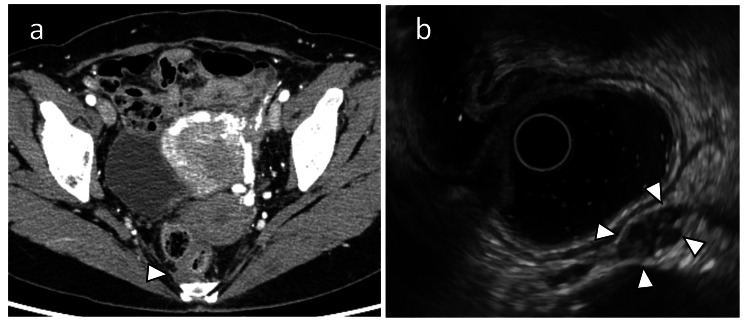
Preoperative CT and transrectal ultrasonography (a) Contrast-enhanced CT scan revealing a poorly defined, low-density mass with no contrast enhancement anterior to the coccyx (arrowhead). (b) Transrectal endoscopic ultrasound showing a 12 mm hypoechoic mass with internal heterogeneity (arrowheads). A biopsy was performed under endoscopic ultrasound guidance; however, no neoplastic lesions were identified, making the diagnosis challenging. CT: computed tomography
